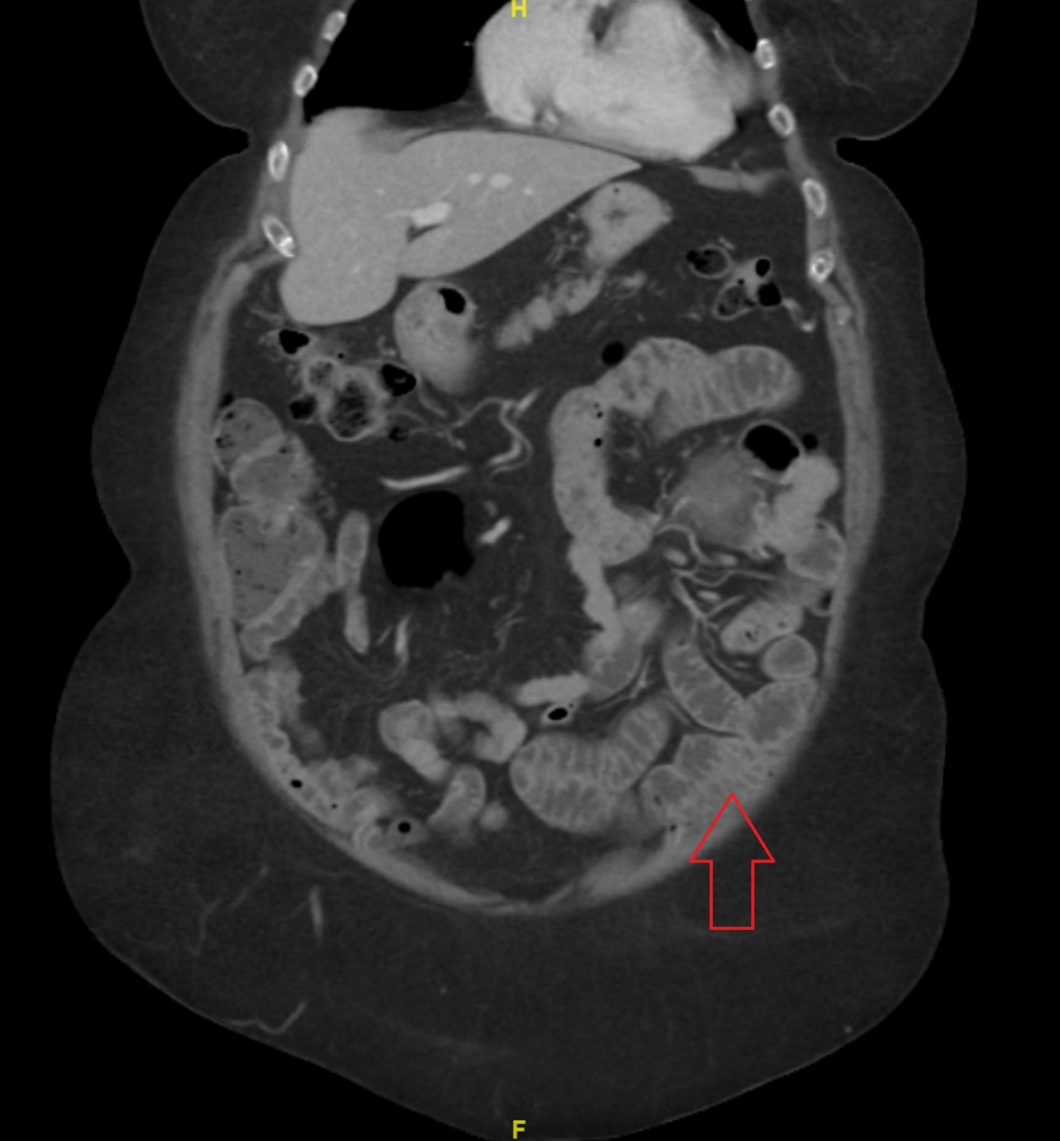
Jejunal diverticulum with surrounding small amount of fluid and inflammatory change (adnominal CT with contrast – coronal view)
